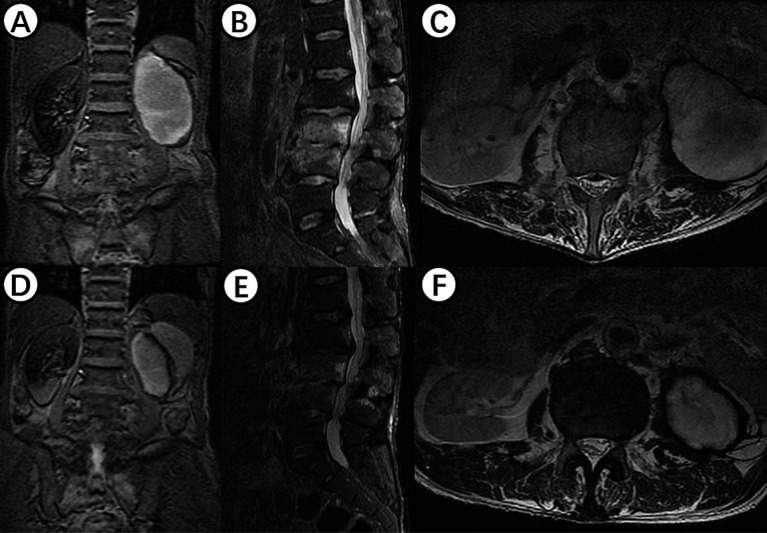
(A–C) Shows MRI examination of the lumbar spine in a patient with hemorrhagic shock, revealing an oval-shaped fluid signal at the lower pole of the left kidney, likely due to hematoma at the surgical site. (D–F) Displays findings from an MRI examination 3 months postoperatively, showing significant reduction of the hematoma signal, with disappearance of inflammatory signals in the L3 and L4 vertebral bodies and intervertebral space.
